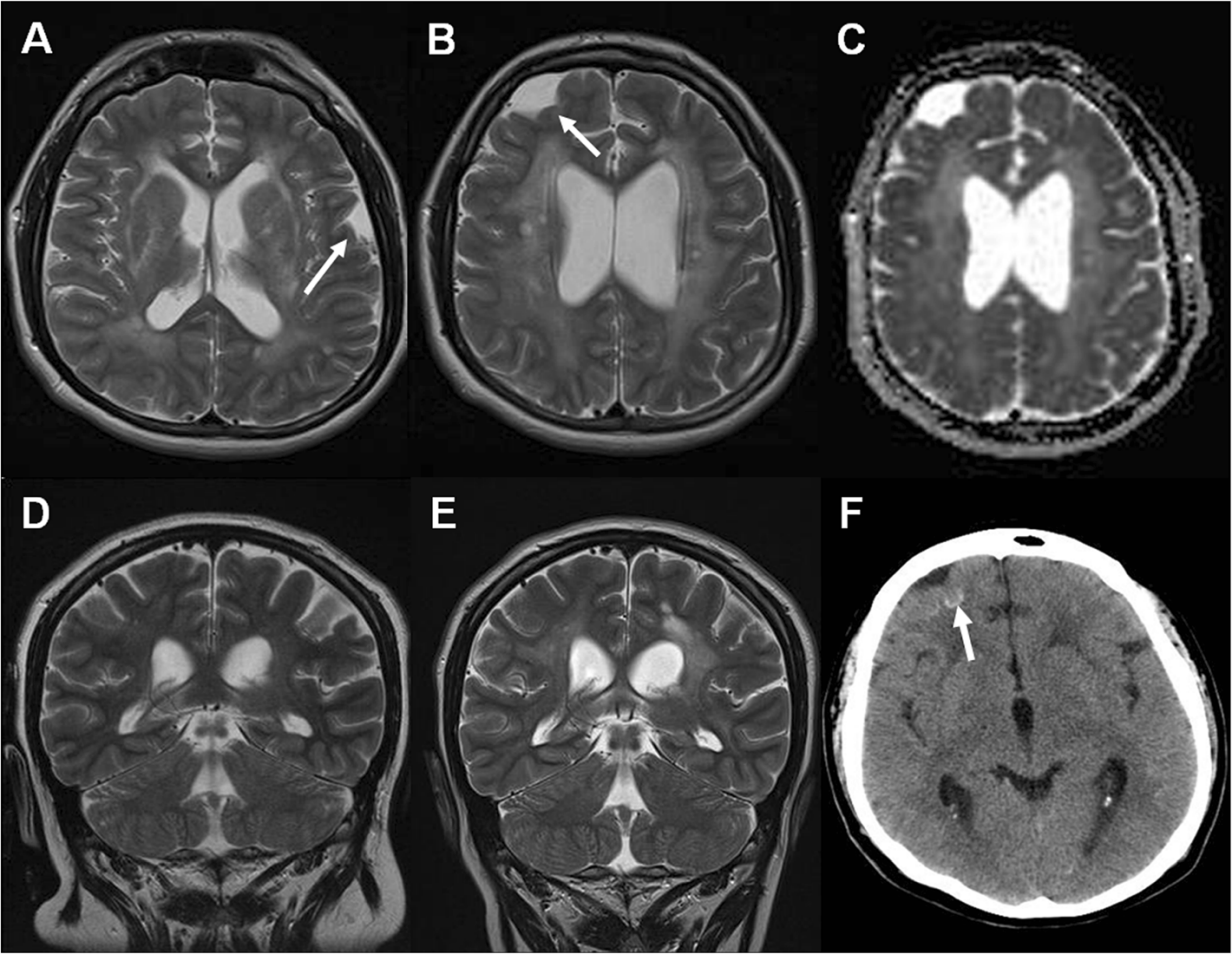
Neuroradiological findings in different examinations. Last magnetic resonance imaging (MRI) study (A and B, axial T2-weighted images; C, apparent diffusion coefficient (ADC) map) shows diffuse hyperintensities in the white matter, patchy involvement of the basal ganglia and thalami, and arachnoid cysts (arrows). ADC is increased, consistent with increased water content in the brain tissue. Comparison of MRI studies obtained 4 years apart (D, E, coronal T2-weighted images) shows progression of the leukoencephalopathy, decrease in size of the subarachnoid spaces along the convexity, and slight enlargement of the ventricles. Recent computed tomography (CT) scan (F) demonstrates tiny subcortical calcifications (arrows). See Additional file 1 for further details.
